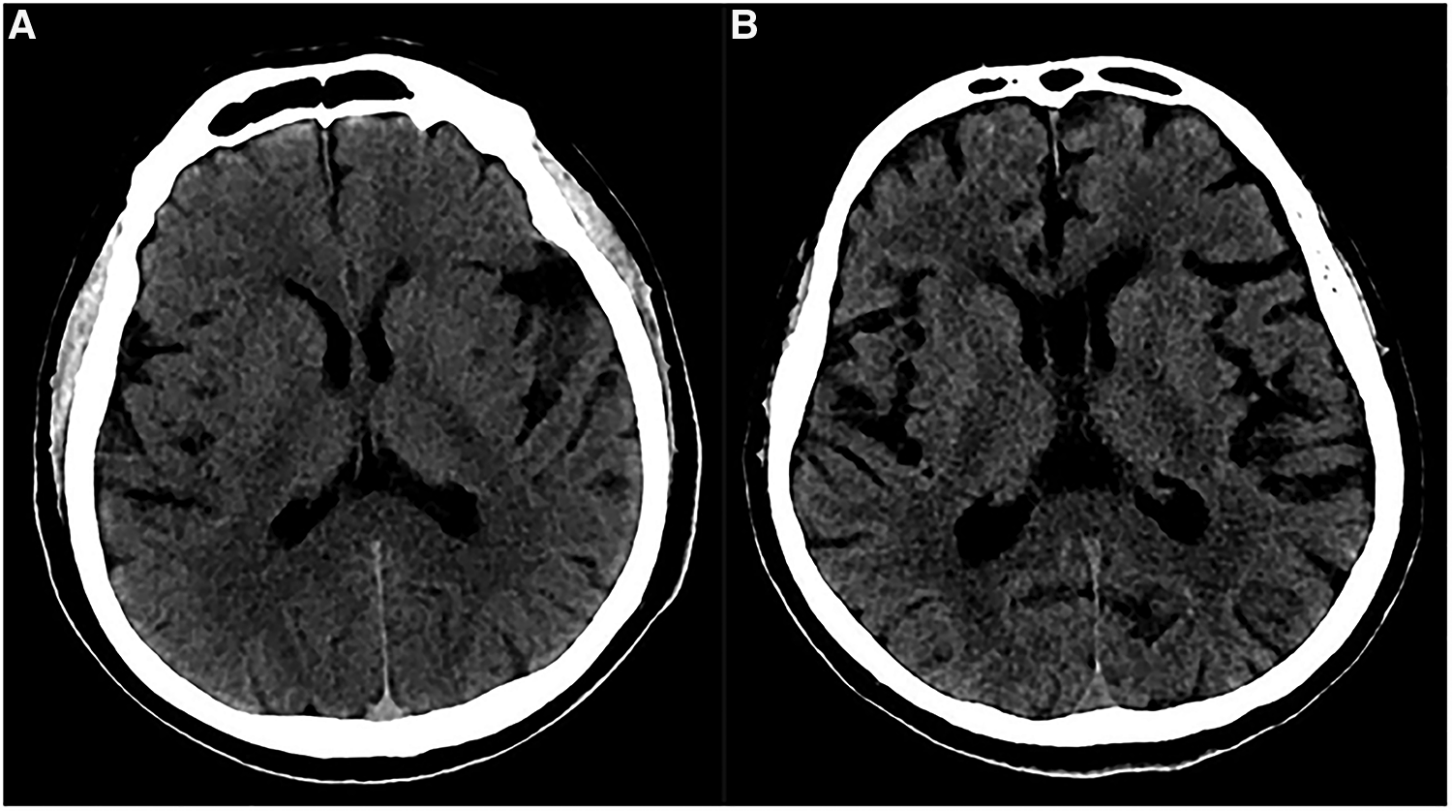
Preoperative imaging of patients with cerebral atrophy. (A) Preoperative CT showed a slight widened Sylvian fissure. (B) Preoperative CT showed more obvious widened cerebral sulcus and fissures as compared to A.
